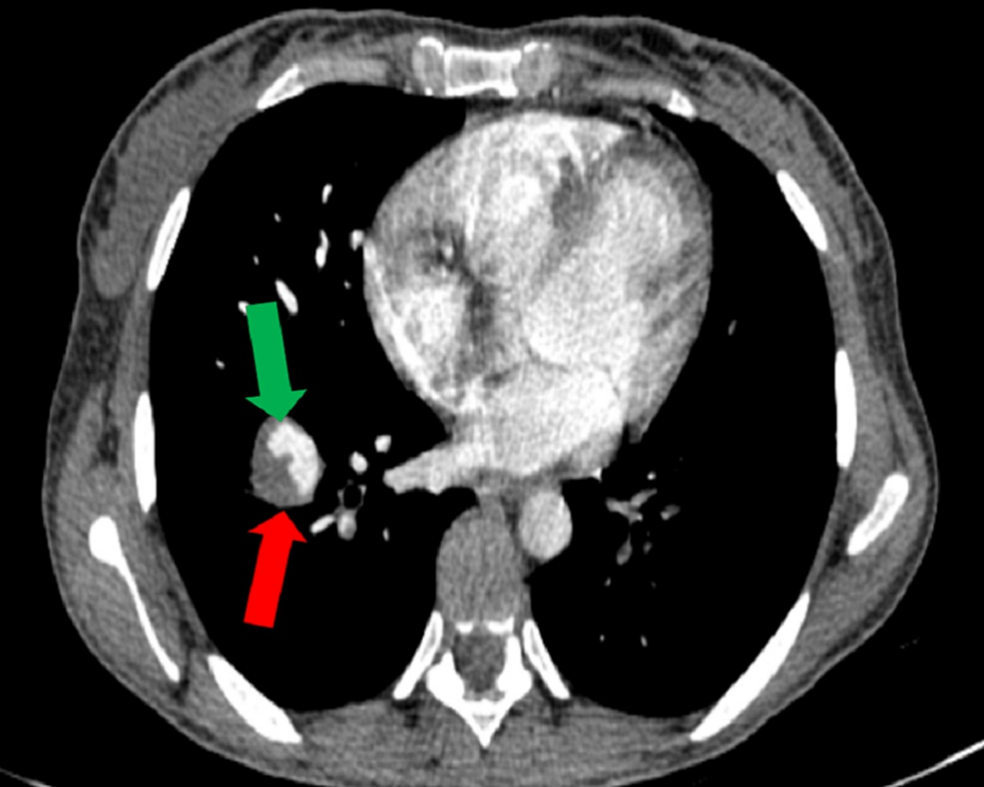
Thorax CT showing pulmonary artery thrombus (green arrow) and aneurysmal dilatation of the right pulmonary artery (red arrow)
